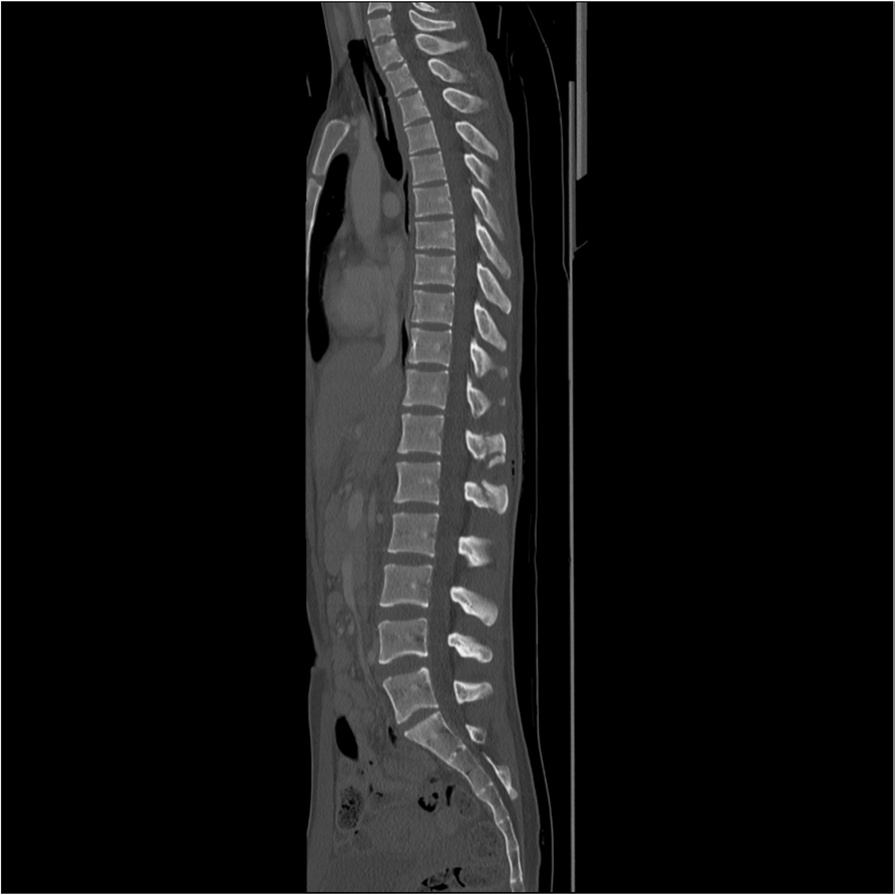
Thoracolumbar spine computed tomography scan: T12 and L1 spinous process fractures
